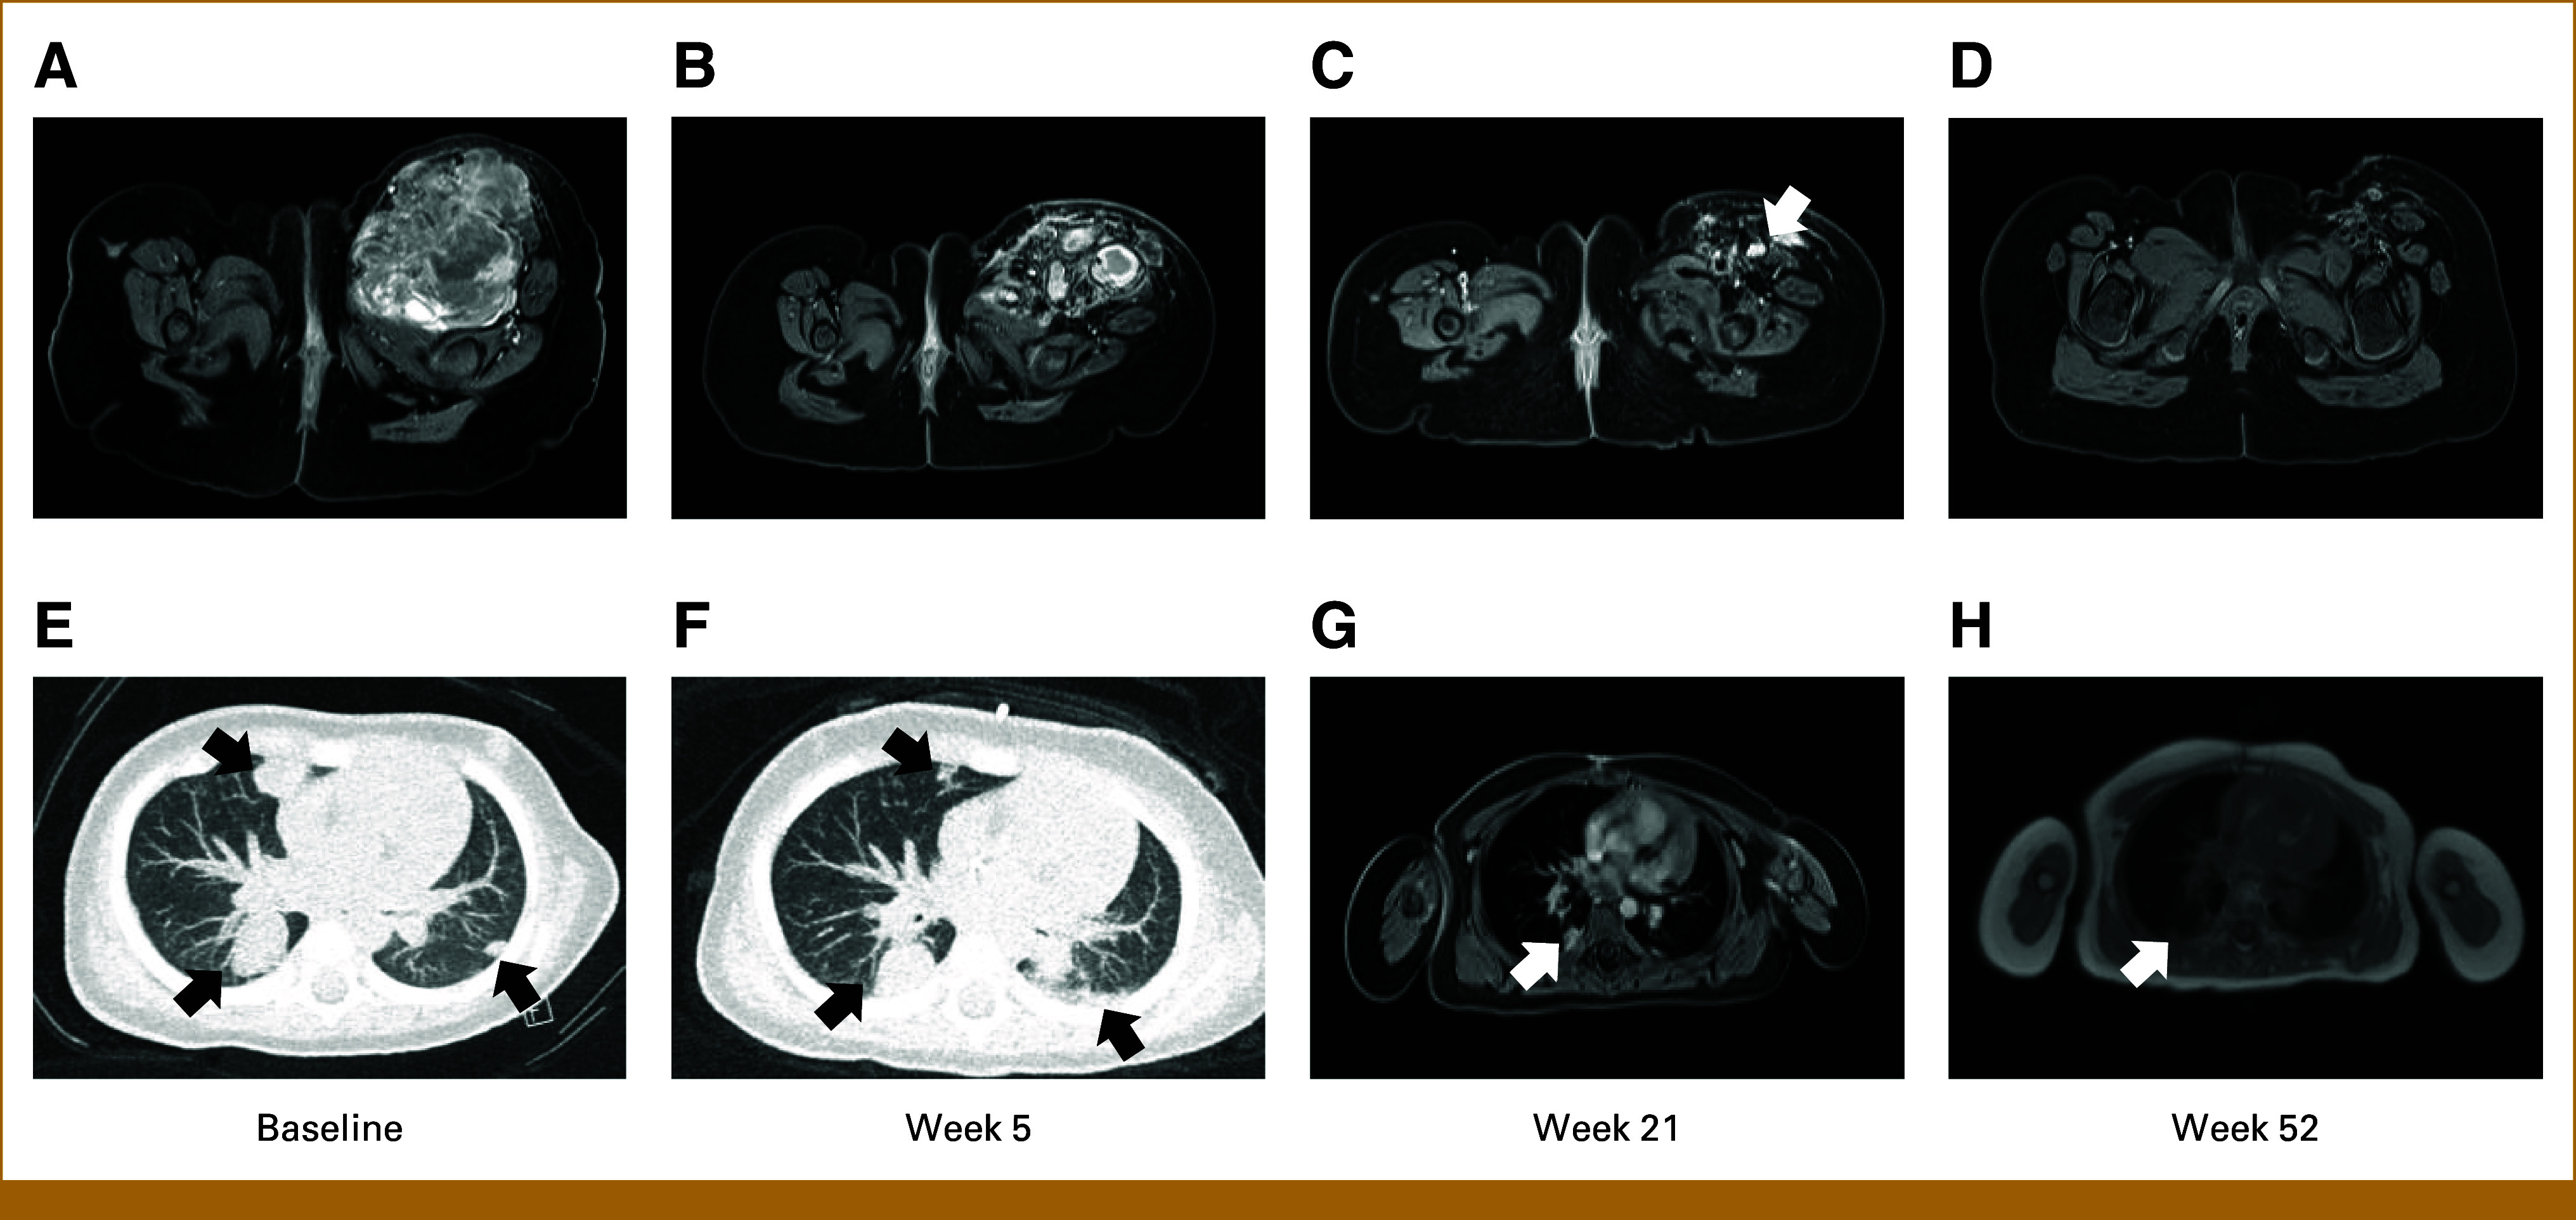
Radiographic response of primary tumor and pulmonary metastases to selpercatinib. Axial T1-weighted MRI images of the proximal left thigh at (A) baseline, (B) week 5, (C) week 21, and (D) week 52, demonstrating marked regression of the primary tumor. By week 21, one of three residual nodules is shown as a representative example (white arrow, C). The pulmonary response: (E and F) axial chest CT scans at baseline and week 5, (G) axial T1-weighted MRI at week 21, and (H) flow-sensitive 3D-MRI at week 52. Pulmonary metastases are indicated by black arrows; fibrotic remodeling at prior metastatic sites is indicated by white arrows. 3D-MRI, three-dimensional magnetic resonance imaging; CT, computed tomography; Flow-3D-MRI, flow-sensitive three-dimensional magnetic resonance imaging; MRI, magnetic resonance imaging; T1-weighted, T1-weighted imaging.
